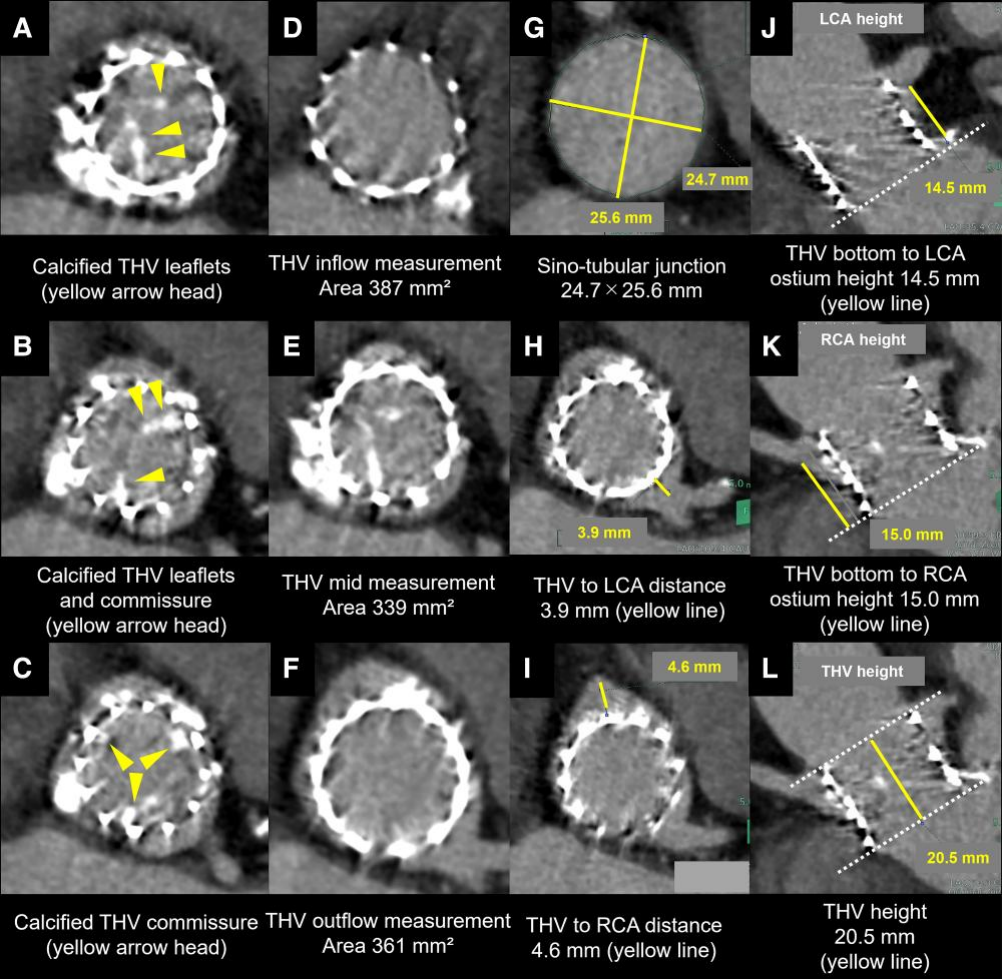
Contrast computed tomography revealed calcified transcatheter heart valve leaflets and commissures *(A–C)*. The transcatheter heart valve area at inflow was 387 mm^2^  *(D)*. The transcatheter heart valve area at mid-portion was 339 mm^2^  *(E)*. The transcatheter heart valve area at outflow was 361 mm^2^  *(F)*. The minimum and maximum sino-tubular junction diameters were 24.7 and 25.6 mm *(G)*, respectively. Distances to the left and right coronary arteries were 3.9 and 4.6 mm, respectively *(H and I)*. The distance of the lowest ostium of the left and right coronary arteries and the bottom of transcatheter heart valve were 14.5 and 15.0 mm, respectively *(J and K)*. The height of transcatheter heart valve was 20.5 mm *(L)*.
